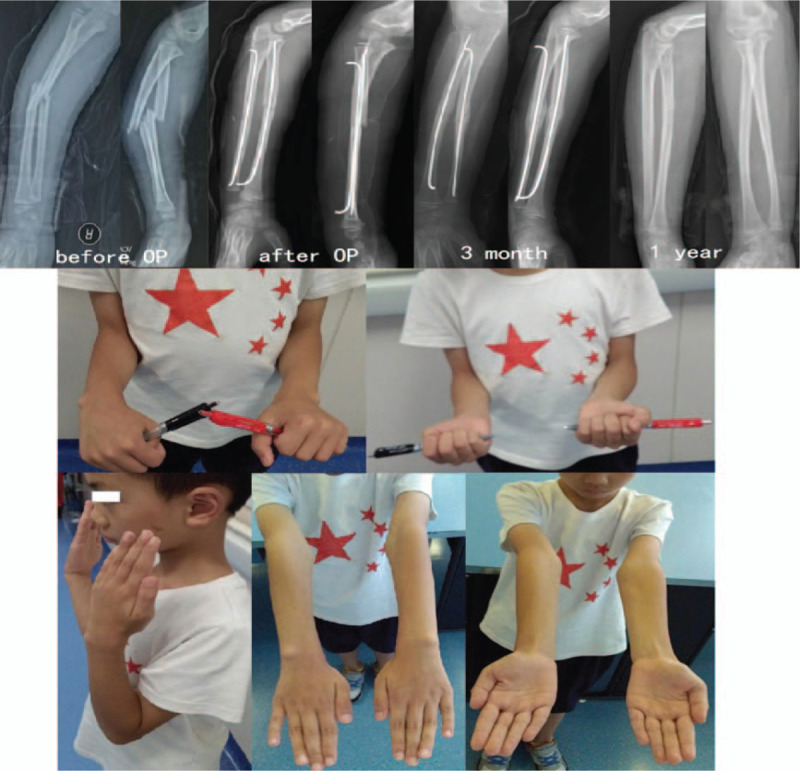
Typical case: roentgen ray imaging and function of forearm.
